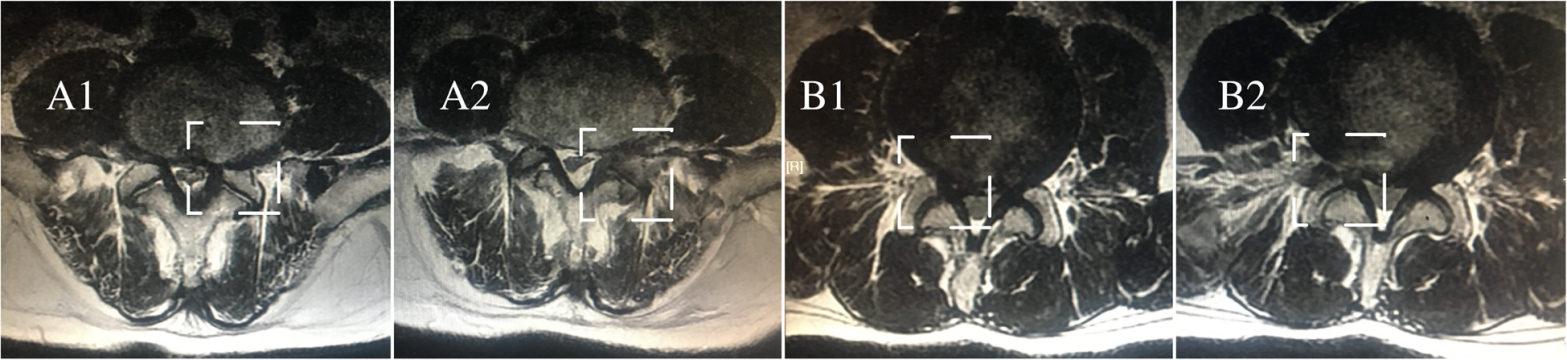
The magnetic resonance imaging (MRI) of patients subjected to PTED with different grades of facetectomy. a. PTED in the left side. b. PTED in the right side. 1. Preoperative data. 2 .Postoperative data
